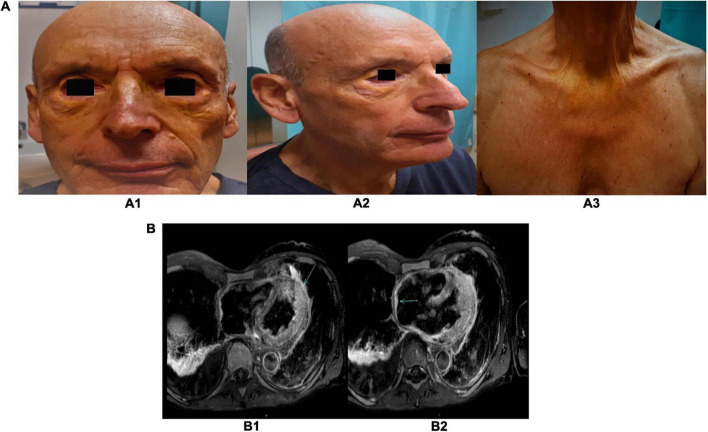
(A) Pictures of the xanthelasmas of the eyelid and thorax. Several yellowish plaque/papules on both upper and lower eyelids, cheeks (A1,A2), and anterior region of the thorax (A3). (B) Cardiac magnetic resonance images. Post-gadolinium T2-weighted sequences, showing a gadolinium-enhanced pericardial nodule (B1) and the pseudotumor of the right atrial roof (B2).
